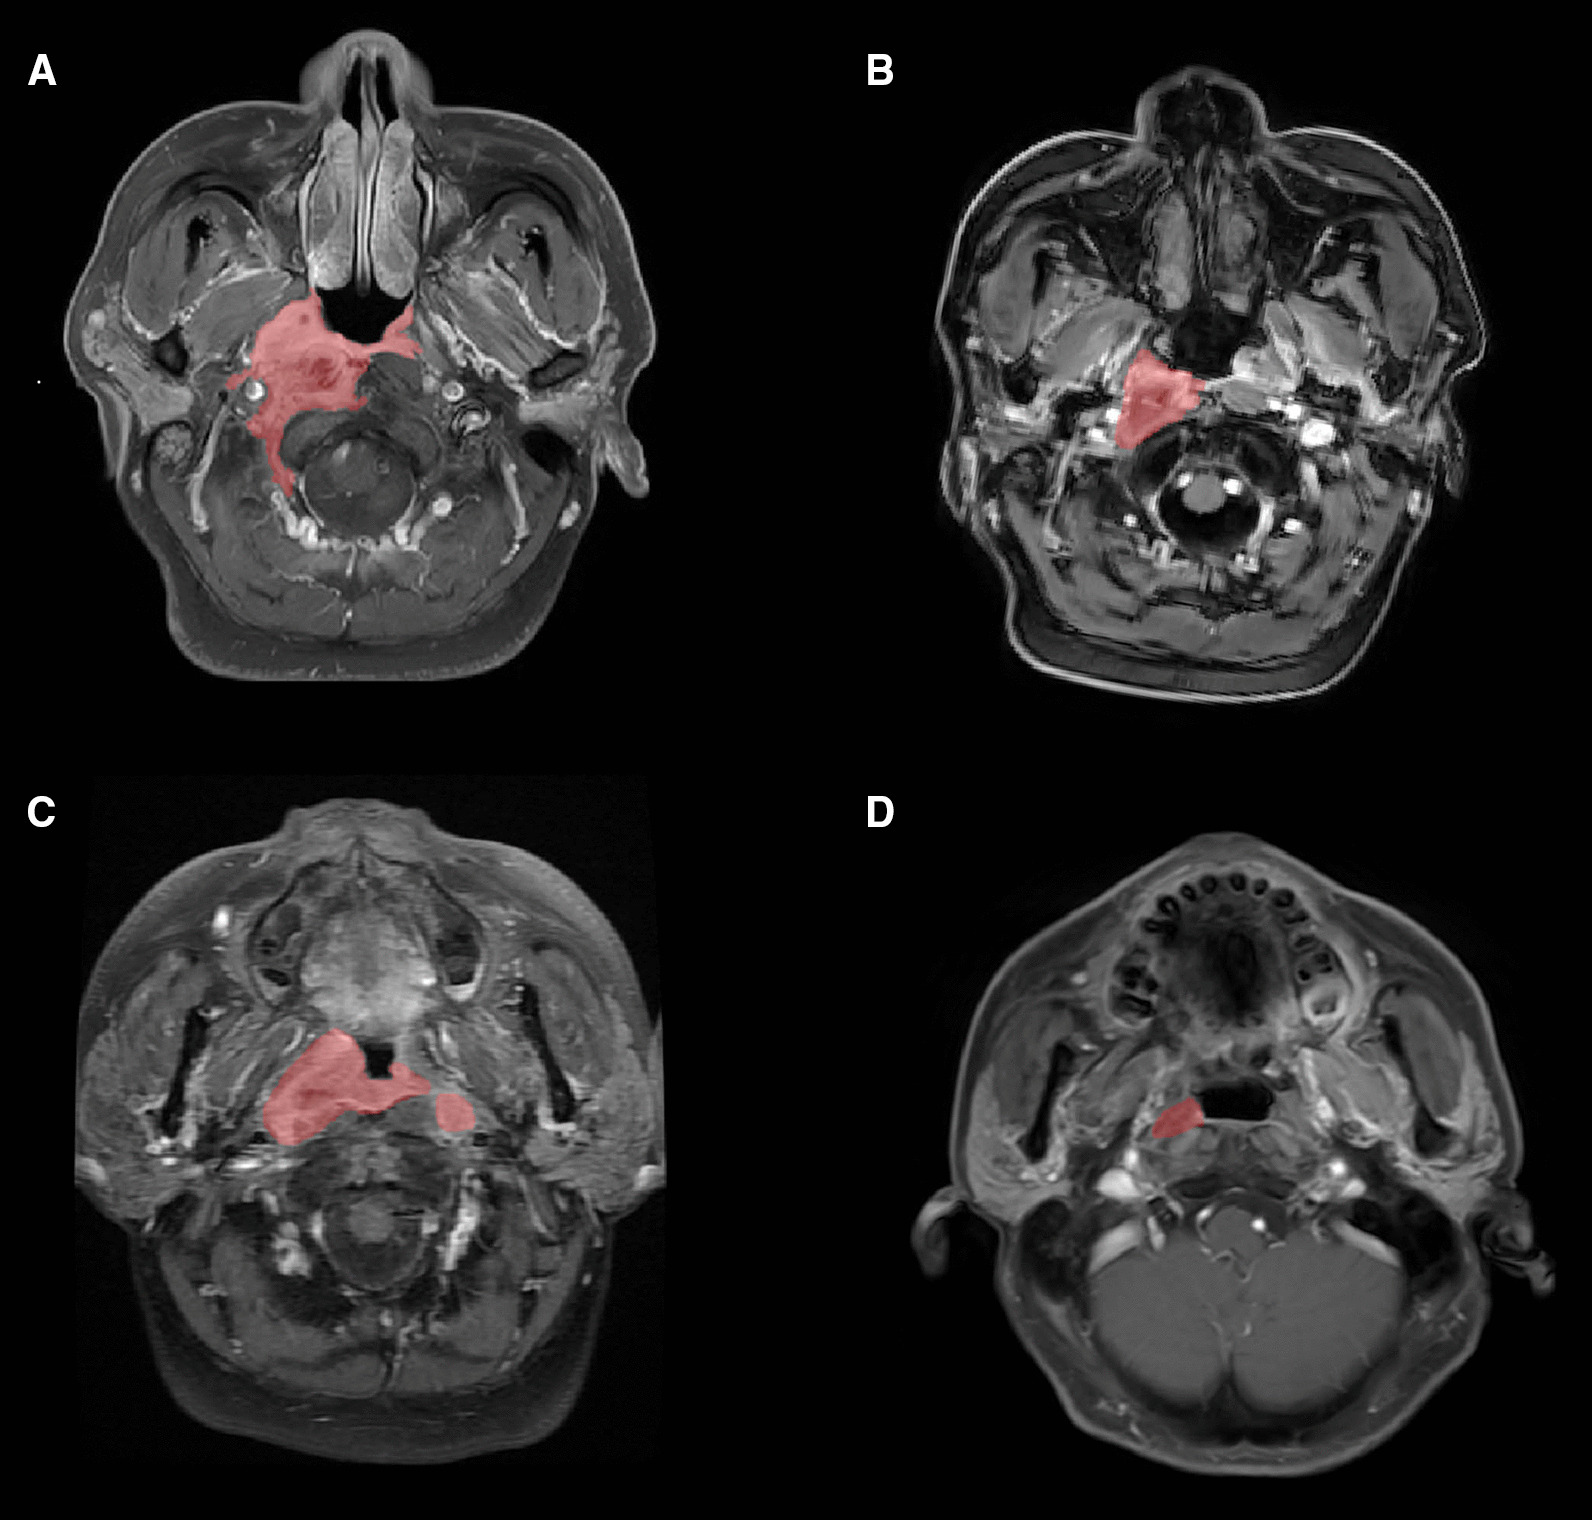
Contrast axial T1-weighted MRI obtained in pre-treatment (A, C) and late-course of IMRT after IC (B, D). ROIs were delineated to indicate the Vpre (A, C, red) and LCV (B, D, red). Case 1: a 43-years of male with T3N2M0 NPC, showing a VRR < 75.1%, from Vpre = 30.2 cm^3^ (A) to LCV = 22.4 cm^3^ (B), suffered from local recurrence at the 28th month of follow-up. Case 2: a 40-years of male with T4N2M0 NPC, showing a VRR > 75.1%, from Vpre = 21.2 cm^3^ (C) to LCV = 1.7 cm^3^ (D), without local recurrence till the final follow-up (at the 81th month). *IMRT* intensity-modulated radiotherapy, *IC* induction chemotherapy, *ROI* tumor regions of interest, *NPC* nasopharyngeal carcinoma, *Vpre* tumor volume before treatment, *LCV* late-course volume, *VRR* volume regression rate
